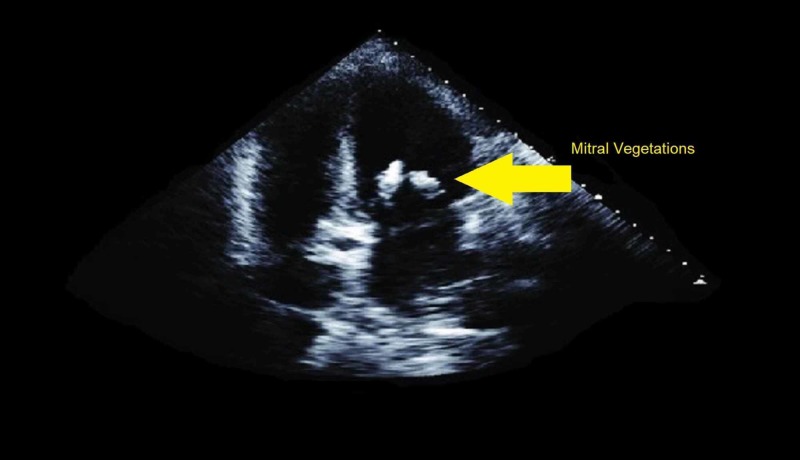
TTE of the patient showing mitral vegetations. TTE, transthoracic echocardiography
